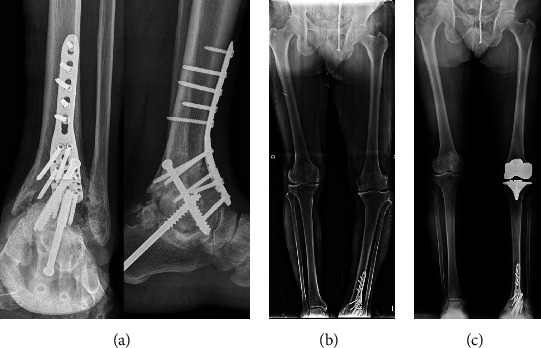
(a, b) The patient underwent tibiotalocalcaneal arthrodesis surgery. (c) Six months after tibiotalocalcaneal arthrodesis surgery, he underwent left total knee arthroplasty (TKA). A standing AP radiograph of the bilateral lower extremities revealed a 1.1° left HKA angle.
